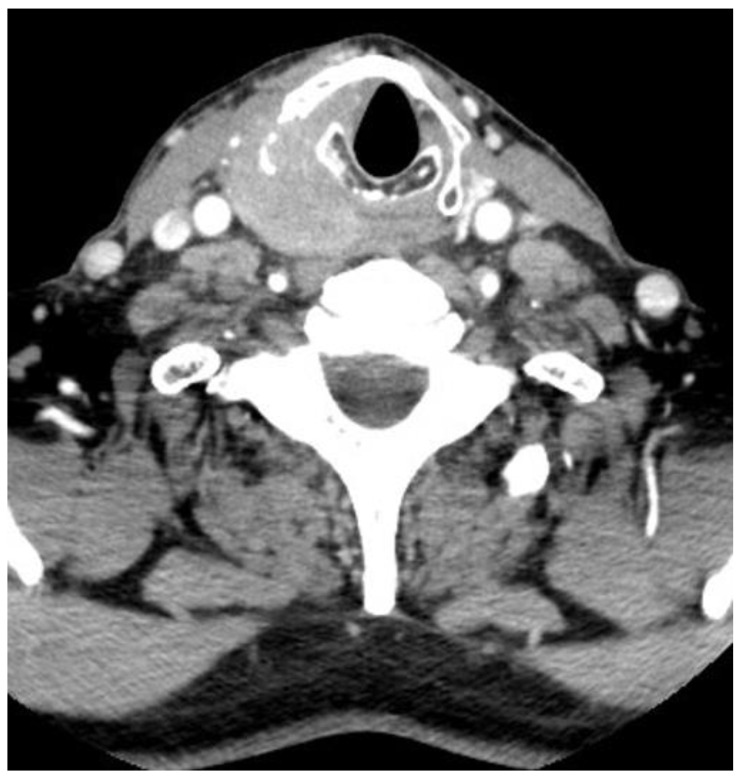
CT scan of a 67-year-old male patient with a T4aN0 hypopharyngeal carcinoma.
